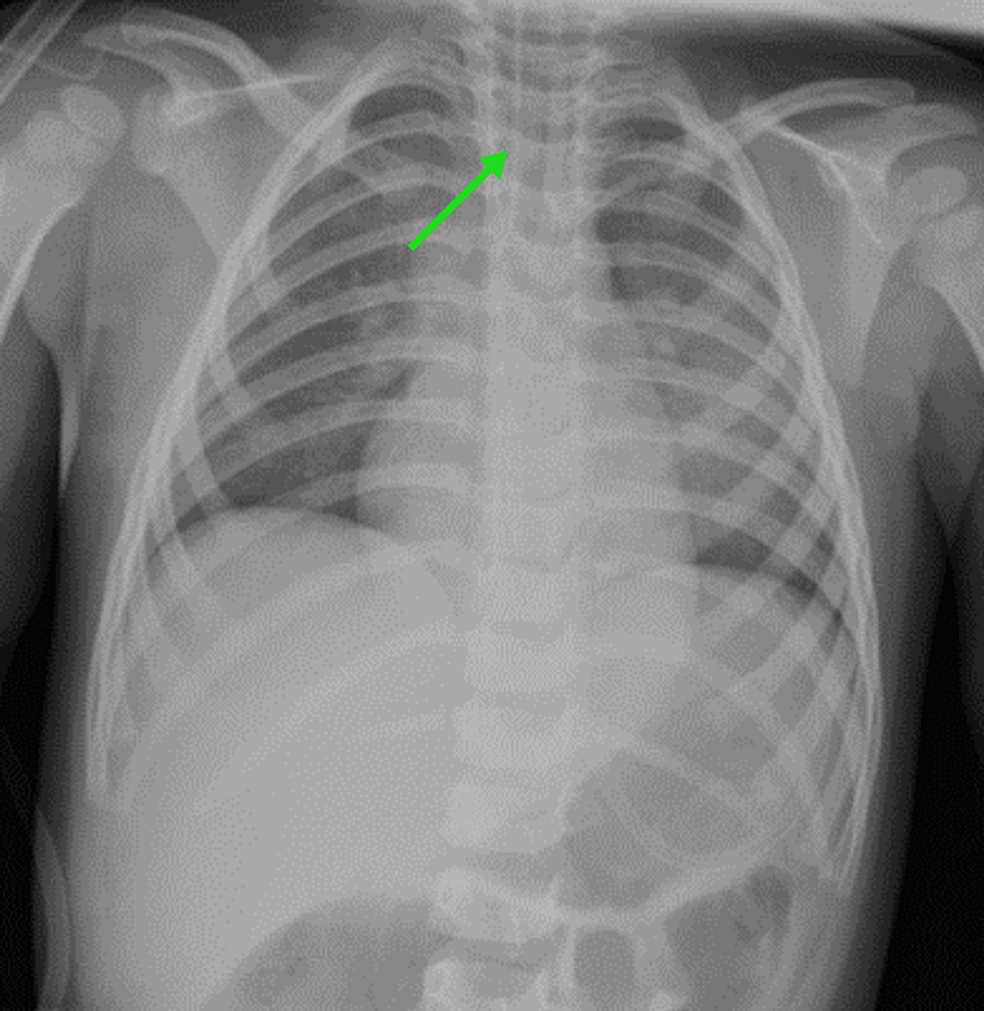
X-ray chest showing the tube in place and a clear lung field
